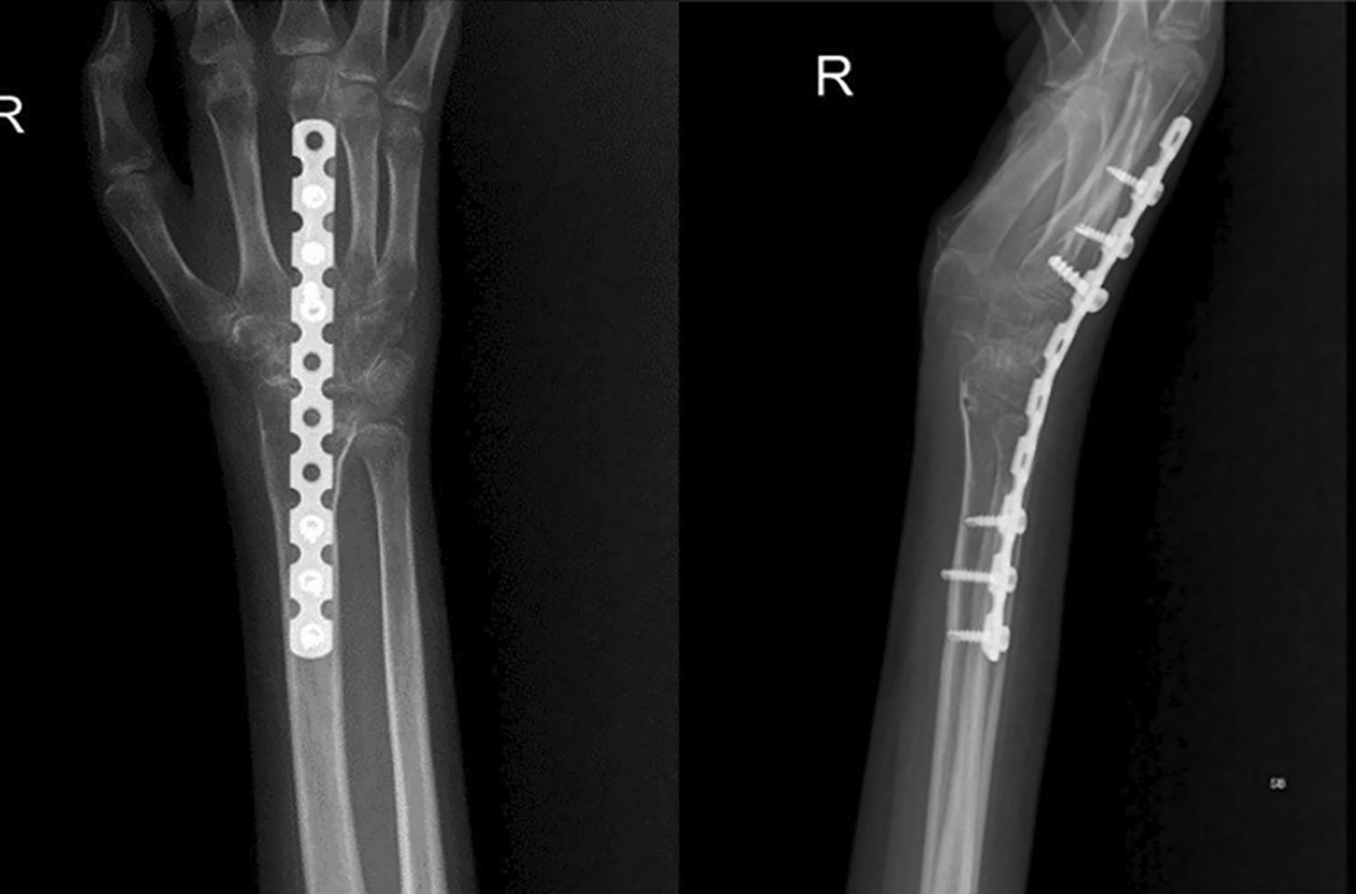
Three years following wrist fusion in a patient with brachial plexus palsy shows union on radiograph with the maintained position with filling up of the donor site
